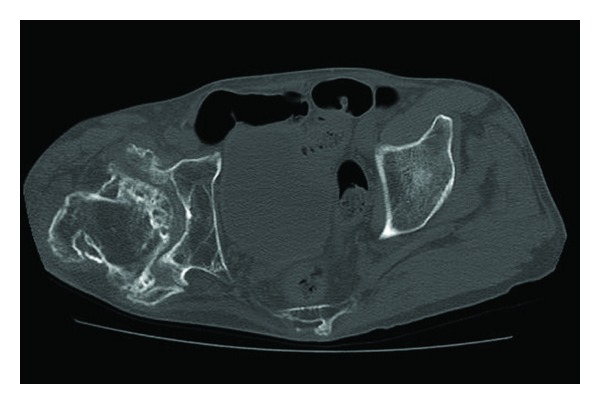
CT imaging around the hip showing marked gluteus muscle atrophy.
